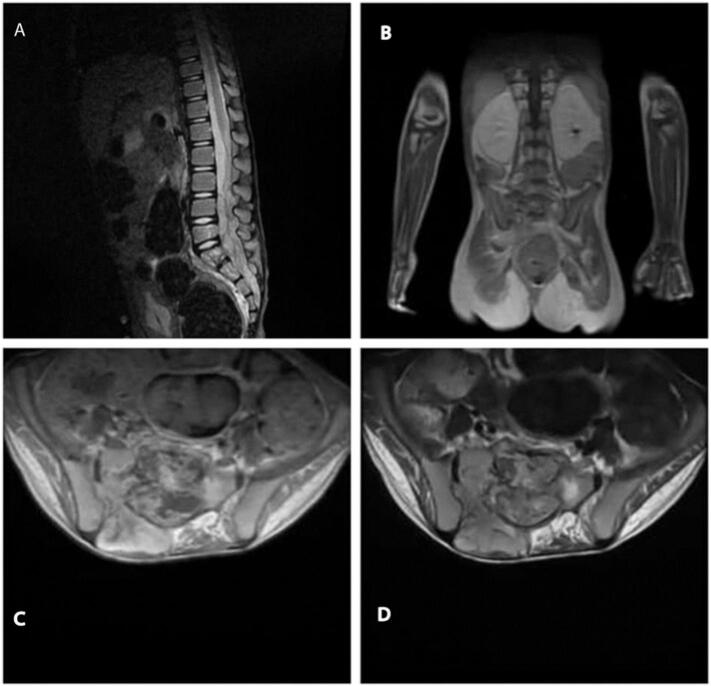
MRI imaging of the spine shows the tumor in (A) the sagittal plane, (B): the coronary plane, (C): T2-weighted transverse plane, and (D): T1-weighted transverse plane.
